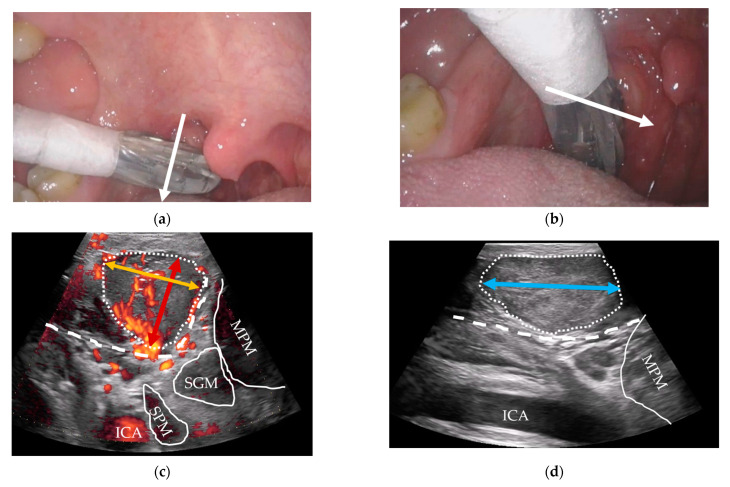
Transoral US of the palatine tonsils: (a) a sheathed US transducer placed on the right palatine tonsil (arrow: swipe direction cranial-to-caudal) in the transverse plane; (b) sagittal plane (arrow: swipe direction lateral-to-medial); (c) transverse US image with Power Doppler of a right palatine tonsil tumor (dotted outline) with underlying constrictor muscle (dashed line), internal carotid artery (ICA), stylopharyngeus muscle (SPM), styloglossus muscle (SGM), and medial pterygoid muscle (MPM). The anteroposterior (red bi-directional arrow) and mediolateral (orange bi-directional arrow) tumor diameters are shown; (d) sagittal US image of the same tumor (dotted outline) with the underlying constrictor (dashed line), ICA, and MPM. In sagittal view, the MPM can be used as a craniolateral landmark. The craniocaudal diameter of the tonsil is shown using a blue bi-directional arrow.
